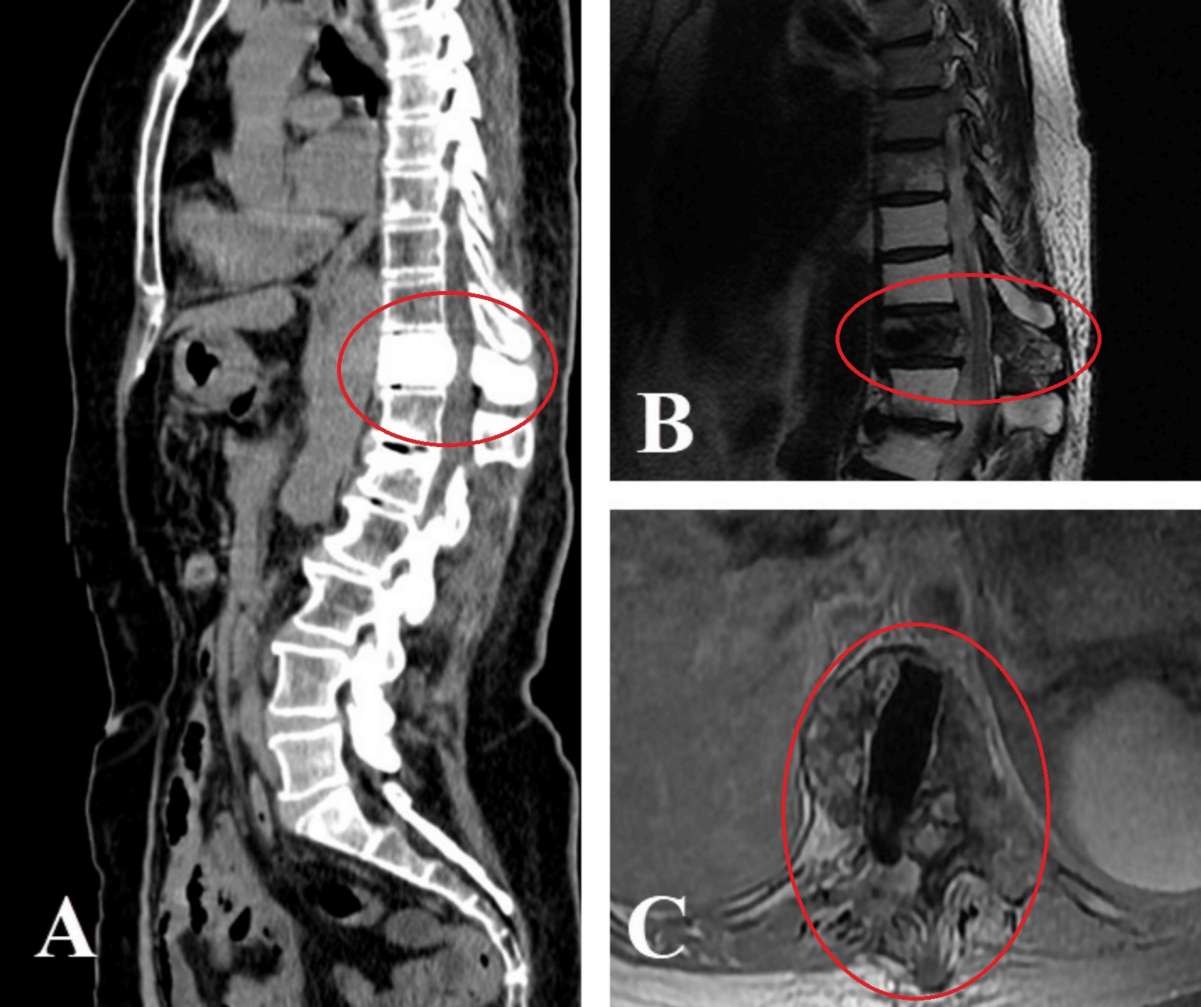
(A) CT scan of the spine shows vertebral augmentation of T11 with methylmethacrylate, preservation of sagittal balance, and decompression of the spinal canal. (B) MRI scan shows passage of cerebrospinal fluid through T11, with T11 appearing hypointense, corresponding to resected bone tissue replaced by methylmethacrylate. (C) Axial section shows the corpectomy site with its path and the area replaced with synthetic material.
